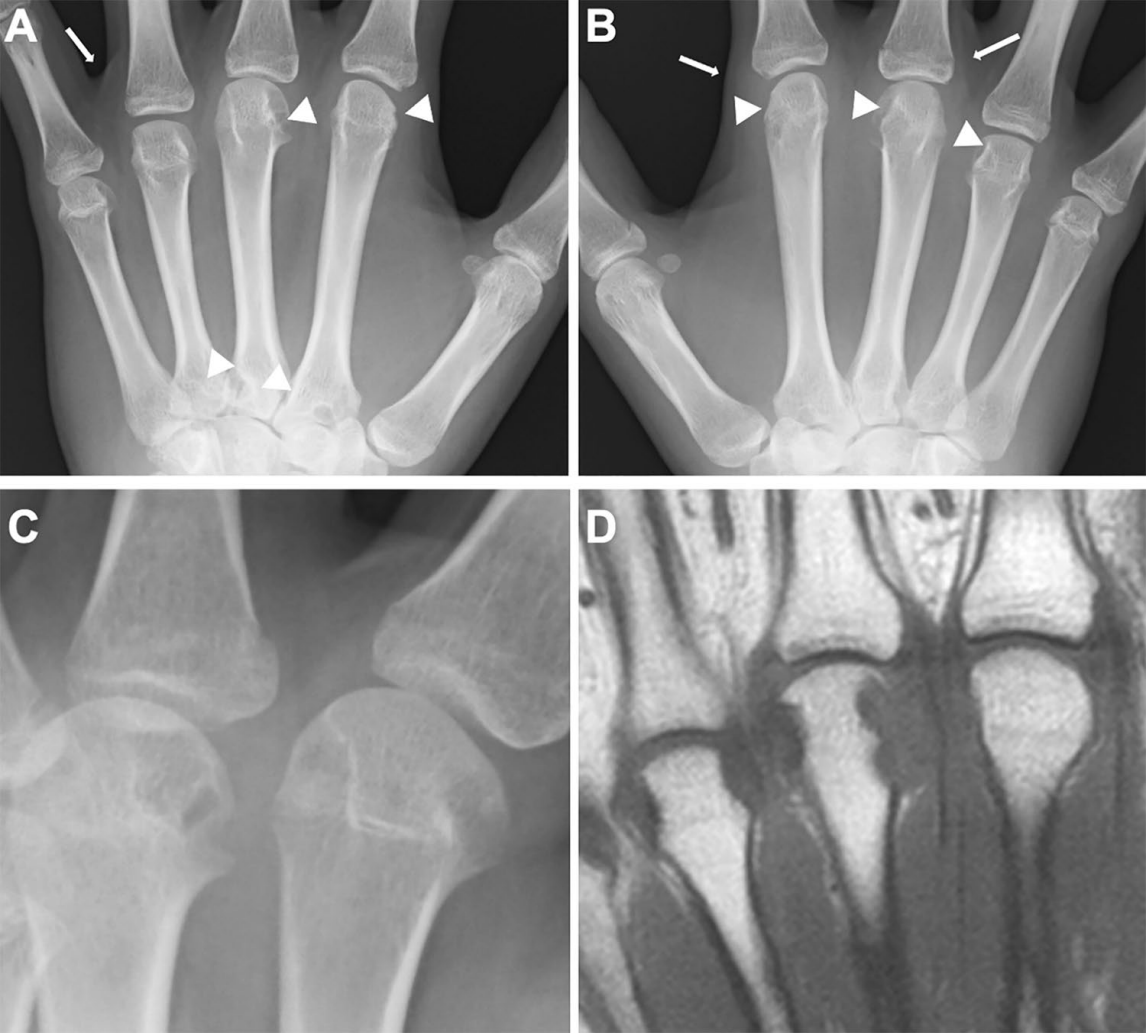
RF positive polyarticular type JIA. A–D. A 14-year-old girl with painful hands. The frontal radiographs of the hands showed marginal erosions of the distal end of the left 2nd and 3rd metacarpals (arrowheads, A), the proximal end of the left 3rd and 4th metacarpals, and the distal end of the right 2nd, 3rd, and 4th metacarpals (arrowheads, B). Periarticular soft tissue swelling was also discernible (arrows, A, B). The close-up view of the left hand (C) and T1-weighted coronal MRI image of the left hand (D) delineated more clearly marginal erosion of the metacarpals
